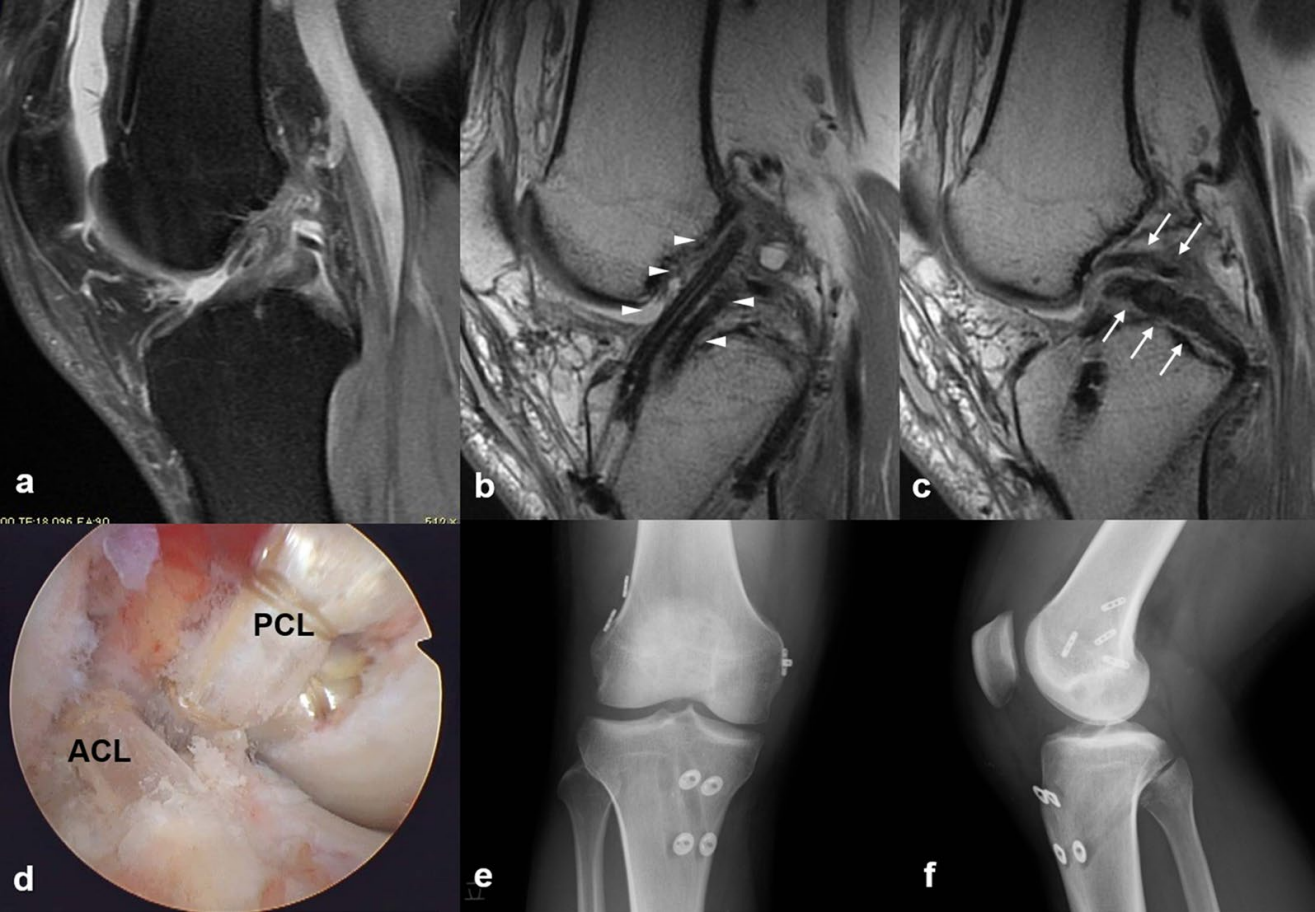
Double-bundle ACL and PCL reconstructions of right knee (26-year-old male judoist). a preoperative MRI showing torn ACL and PCL; b, c postoperative MRI showing reconstructed ACL (arrow heads) and PCL (arrows), d arthroscopic view of double-bundle ACL and PCL reconstructions, and e, f postoperative radiograph. ACL: anterior cruciate ligament; PCL: posterior cruciate ligament; MRI: magnetic resonance imaging
